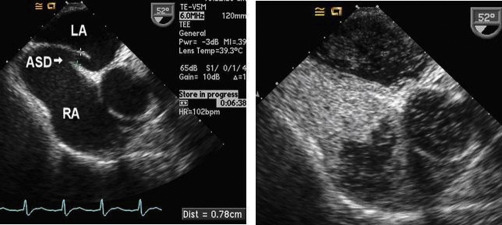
(a) Transesophageal echocardiography showing a large atrial septal defect (ASD) between the left atrium (LA) and right atrium (RA). (b) Following intravenous injection of agitated saline, contrast is seen crossing the ASD into the left side.
